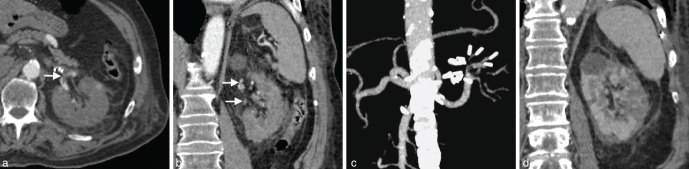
(a) Arterial-phase contrast-enhanced CT axial image shows a filling defect in the distal main trunk of the left renal artery corresponding to thromboembolic material (arrow). (b) Extension to both polar superior and inferior renal artery branches is also seen in the coronal view (arrows), the latter being completely occluded. (c) Three-dimensional maximum intensity projection reconstructed image better obviates the features previously described. (d) Large wedge-shaped areas of non-enhancing renal parenchyma are clearly depicted in the nephrographic phase of the same contrast-enhanced CT scan, consistent with extensive infarction.
